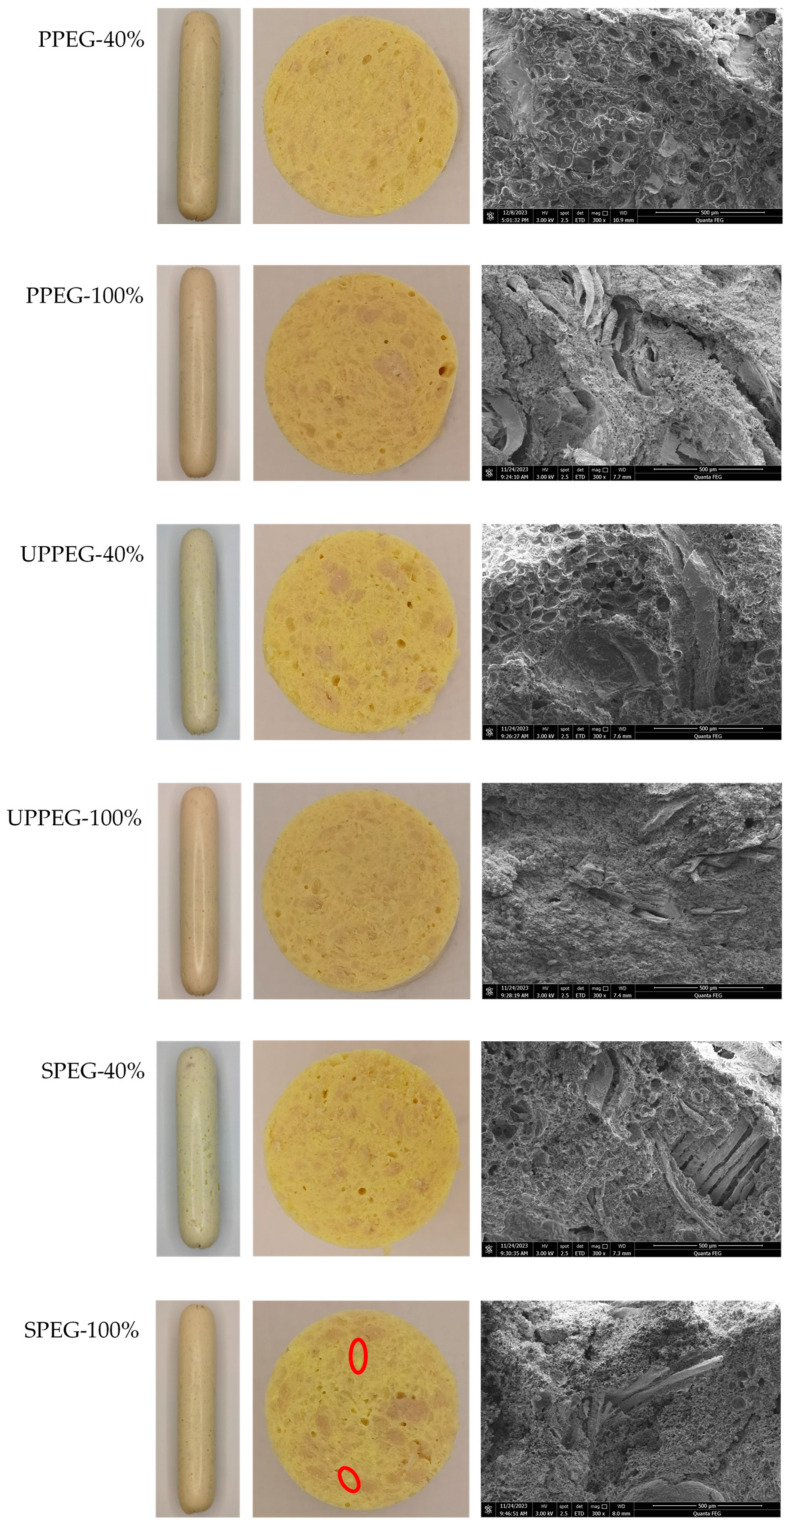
Appearance, cross-sections, and SEM images of sausages after replacing pork backfat with different protein emulsion gels. Control: without emulsion gel; PPEG: peanut protein emulsion gel; UPPEG: ultrasound-modified peanut protein emulsion gel; SPEG: soy protein emulsion gel. SEM images represent cross-sections of freeze-dried sausages. The bars in the SEM images indicate a length of 500 μm. Holes and gel clumps in the surface (cross-section) are indicated by red arrows and circles, respectively.
